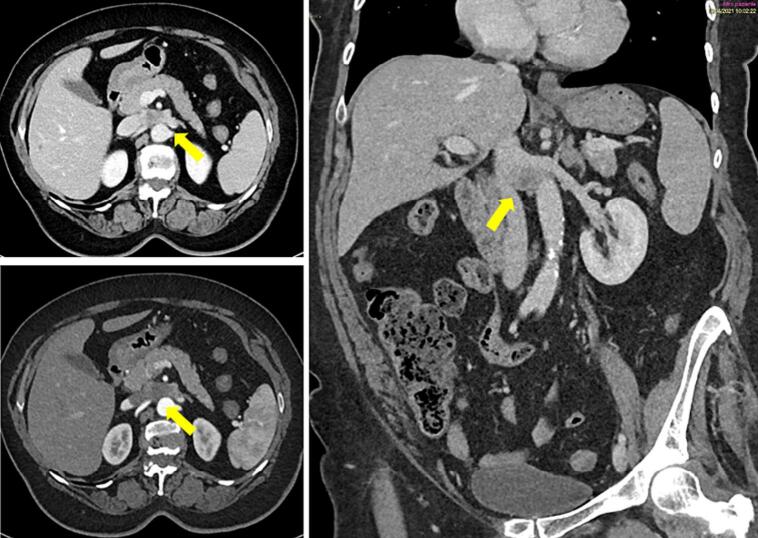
Preoperative CT-scan show a small lesion arising from the left renal vein.
